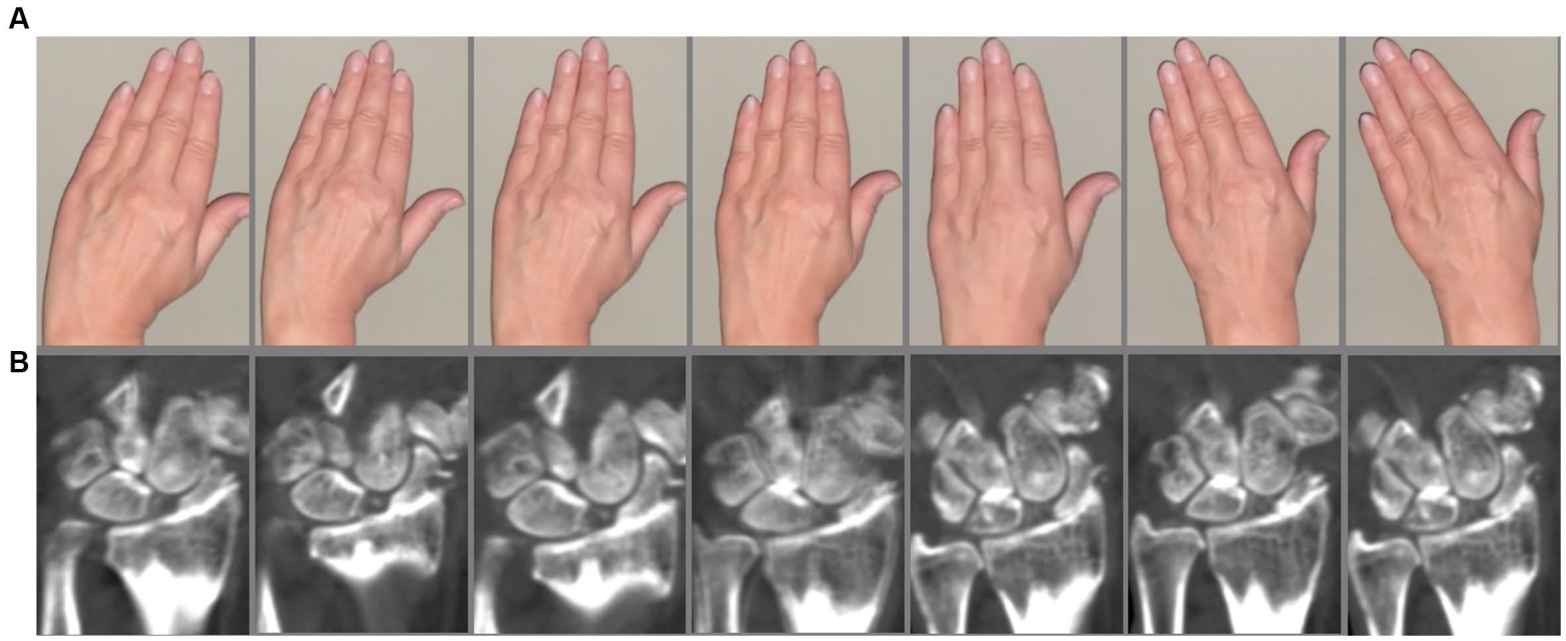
4D-CT scan. (A) Sequences of the instruction video. (B) Patient with SLAC wrist. Static SL ligament instability with severe osteoarthritis.
